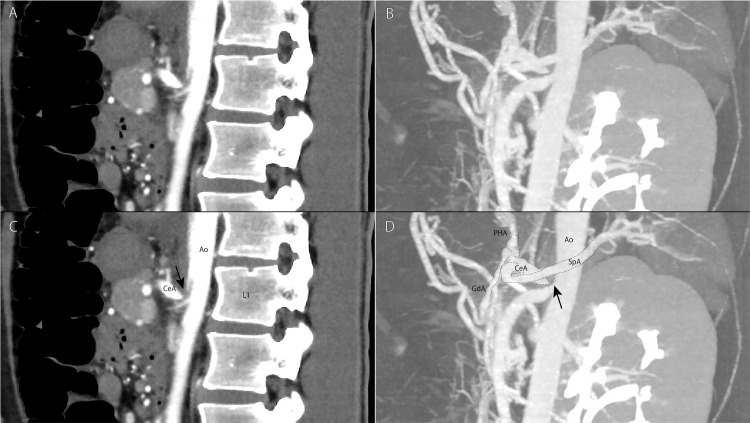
Computed tomography images of the median arcuate ligament Sagittal view (A and C) and 3D re-structured (B and D) contrast-enhanced computed tomography images show the presence of median arcuate ligament syndrome. The black arrow indicates narrowing of the celiac artery. A characteristic hooked appearance is observed owing to the proximal narrowing of the celiac artery with inferior displacement due to indentation by the median arcuate ligament. Ao: aorta; CeA: celiac artery; L: lumber vertebra; GdA: gastroduodenal artery; PHA: proper hepatic artery; SMA: superior mesenteric artery; SpA: splenic artery
